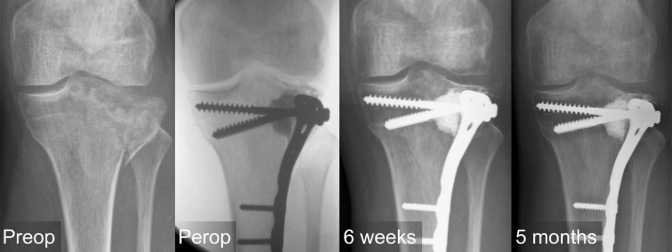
Split depression fracture (Schatzker II) of the lateral tibial plateau in a 62-year-old man. Follow-up radiographs showed no loss of reduction.
